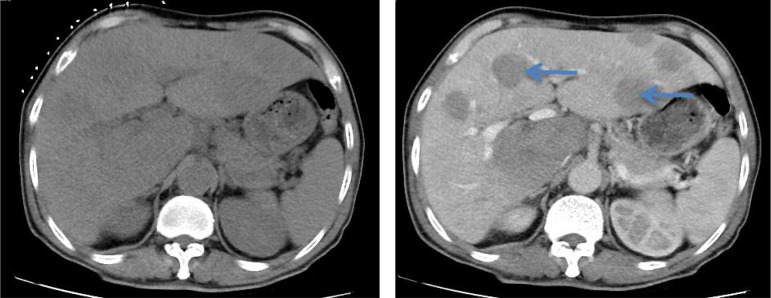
Abdominal enhanced CT scan on November 26, 2021 identified multiple hepatic lesions. The blue arrow indicates a liver mass lesion.
